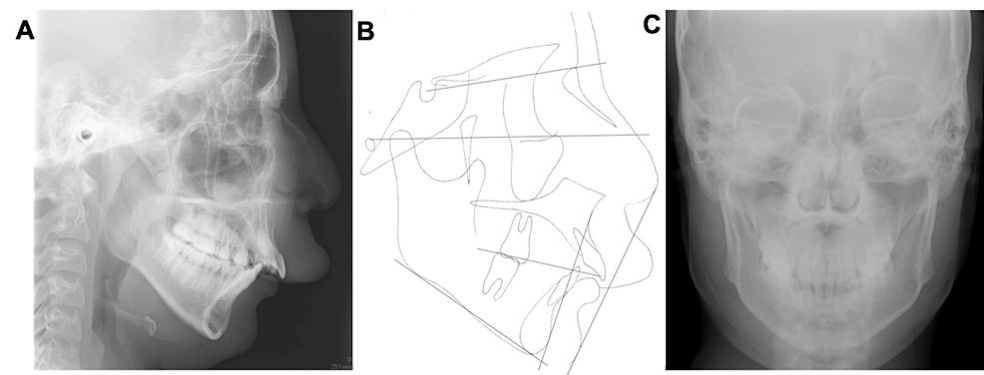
Pretreatment evaluation. (A) Lateral cephalometric radiograph. (B) Original tracing of lateral view. (C) Frontal Cephalometric radiograph.
